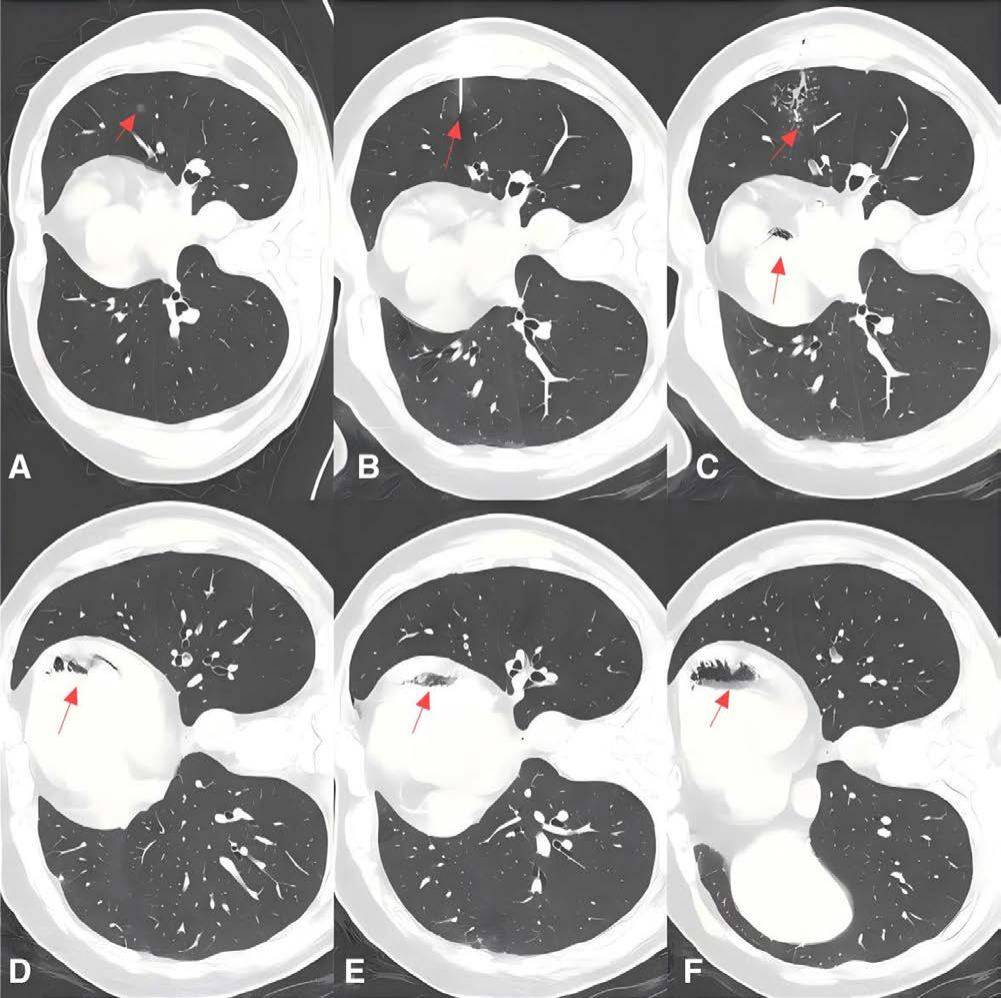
Chest CT on February 25, 2025 (day 0). (A) Baseline scan showing an 8-mm pure ground-glass nodule (white arrow) in the lingular segment of the left upper lobe. (B) Axial slice obtained immediately after hook-wire deployment demonstrates accurate anchoring of the metallic tip (white arrowhead). (C–F) Consecutive slices from the same post-deployment series: scant parenchymal hemorrhage and a small pneumothorax surround the wire track (white arrowheads), while multiple intravascular gas collections are already visible in the aortic root (C), branches of the left superior pulmonary vein (D), the left atrial appendage (E) and the apex and lateral wall of the left ventricle (F) (red arrows). CT = computed tomography.
